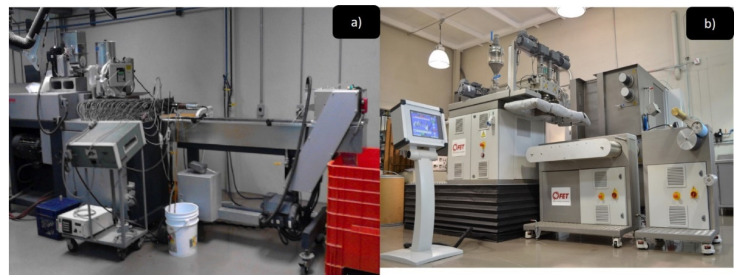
Images of extruders used in to manufacture nonwoven fabric: (a) twin-screw extruder connected to a homemade ultrasonic generator; and (b) fiber extrusion technology (FET-UK) pilot machine.
